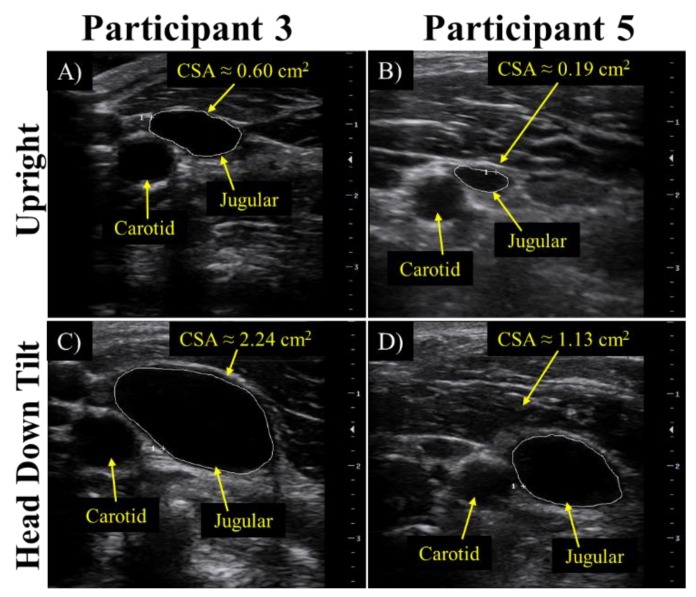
For the 30 min head down tilt at 15°, cross-sectional area data was obtained from ultrasound of the jugular vein as seen in upright position for (A) participant 3 and (B) participant 5, and for head down tilt for (C) participant 3 and (D) participant 5.
